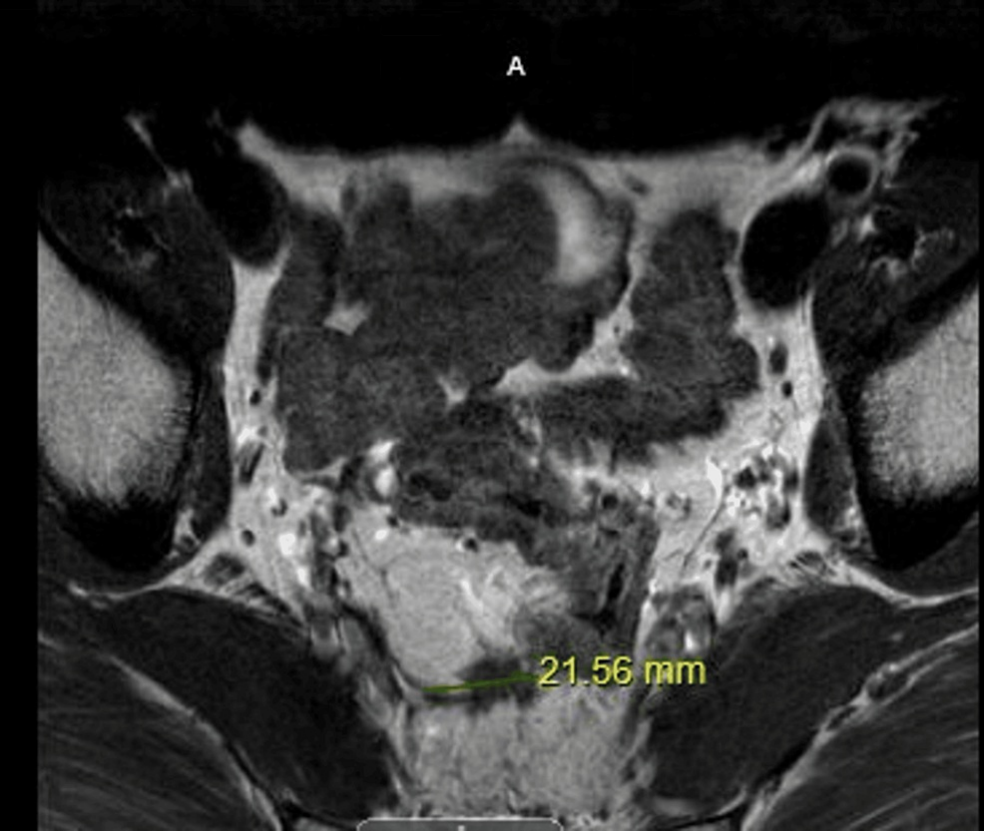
MRI with IV contrast, T2 axial view The image shows 4.3 cm upper to mid-rectal carcinoma with adjacent diffuse posterior perirectal tumor infiltration and posterolateral mesorectal lymphadenopathy MRI: magnetic resonance imaging
